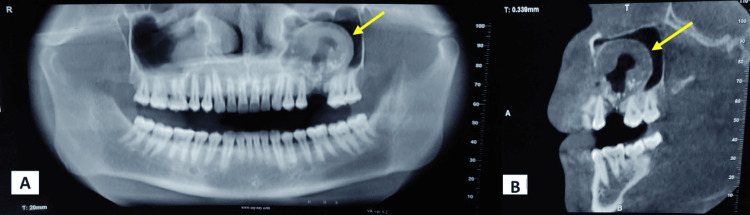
CBCT showing a corticated unilocular radiolucent lesion with a thickened lining and radiopaque flecks, originating from the floor of the left maxillary antrum CBCT, cone beam CT
